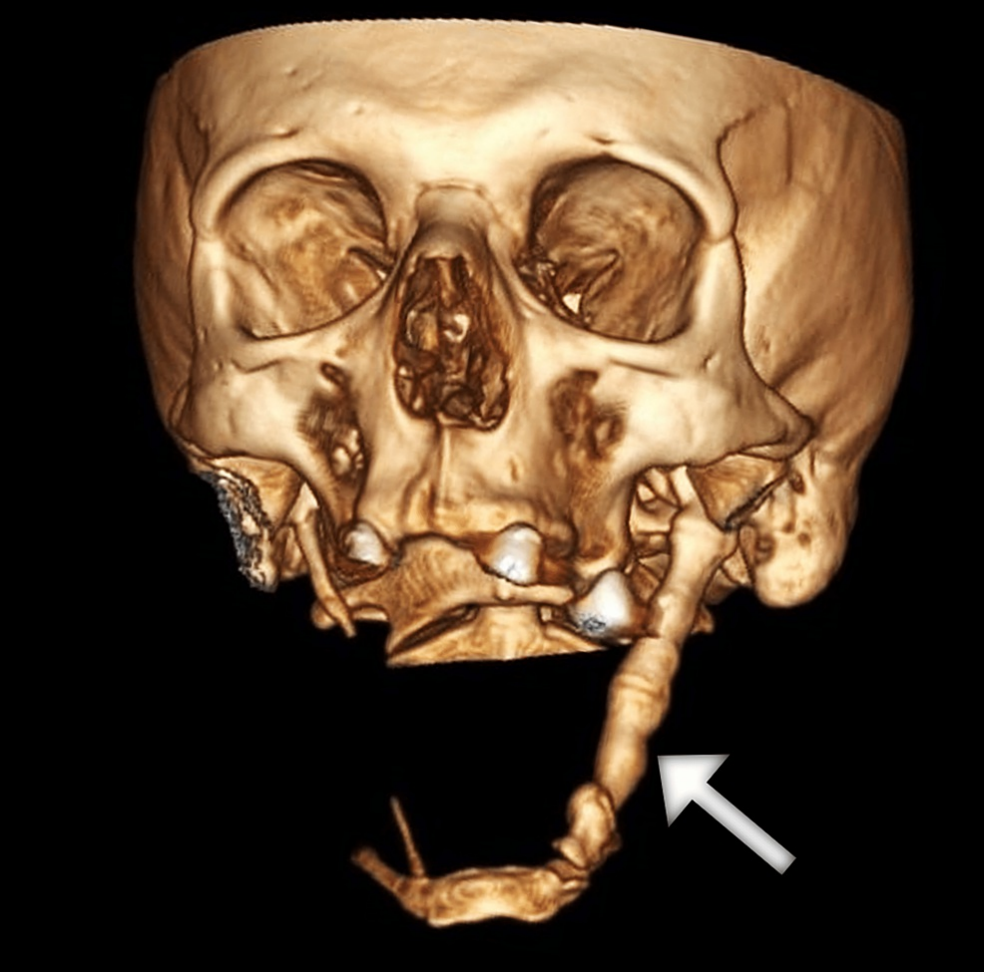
Three-dimensional CT image of the head shows a prolonged left styloid process (arrow). CT: computed tomography
